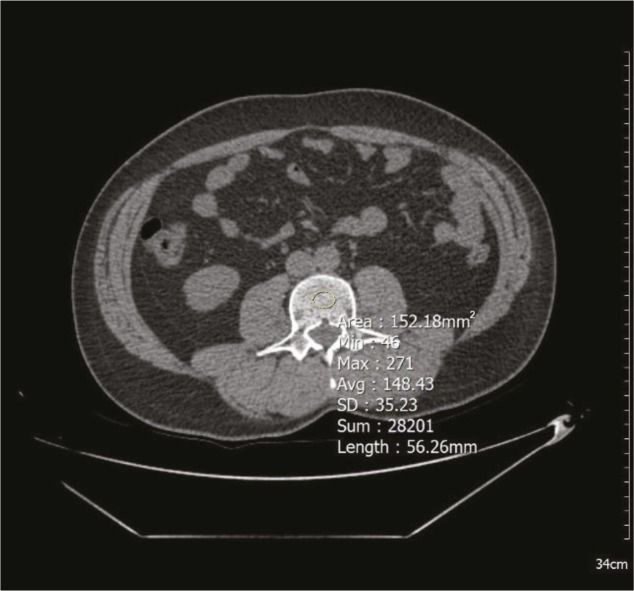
The vertebral density measurement is shown on a transverse CT image. 148 HU, normal vertebral density.
